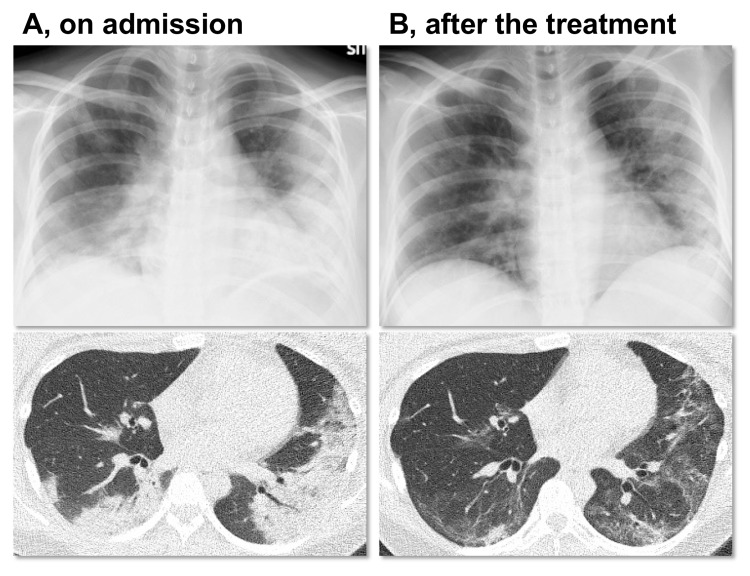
Imaging findings of COVID-19 pneumonia (A) Chest X-ray (upper) and non-contrast computed tomography (CT) (lower) showed multifocal ground-glass opacities with consolidation in the bilateral lungs on admission. (B) Findings of chest X-ray (upper) and non-contrast CT (lower) ameliorated after the treatment of COVID-19 (on day 19).
